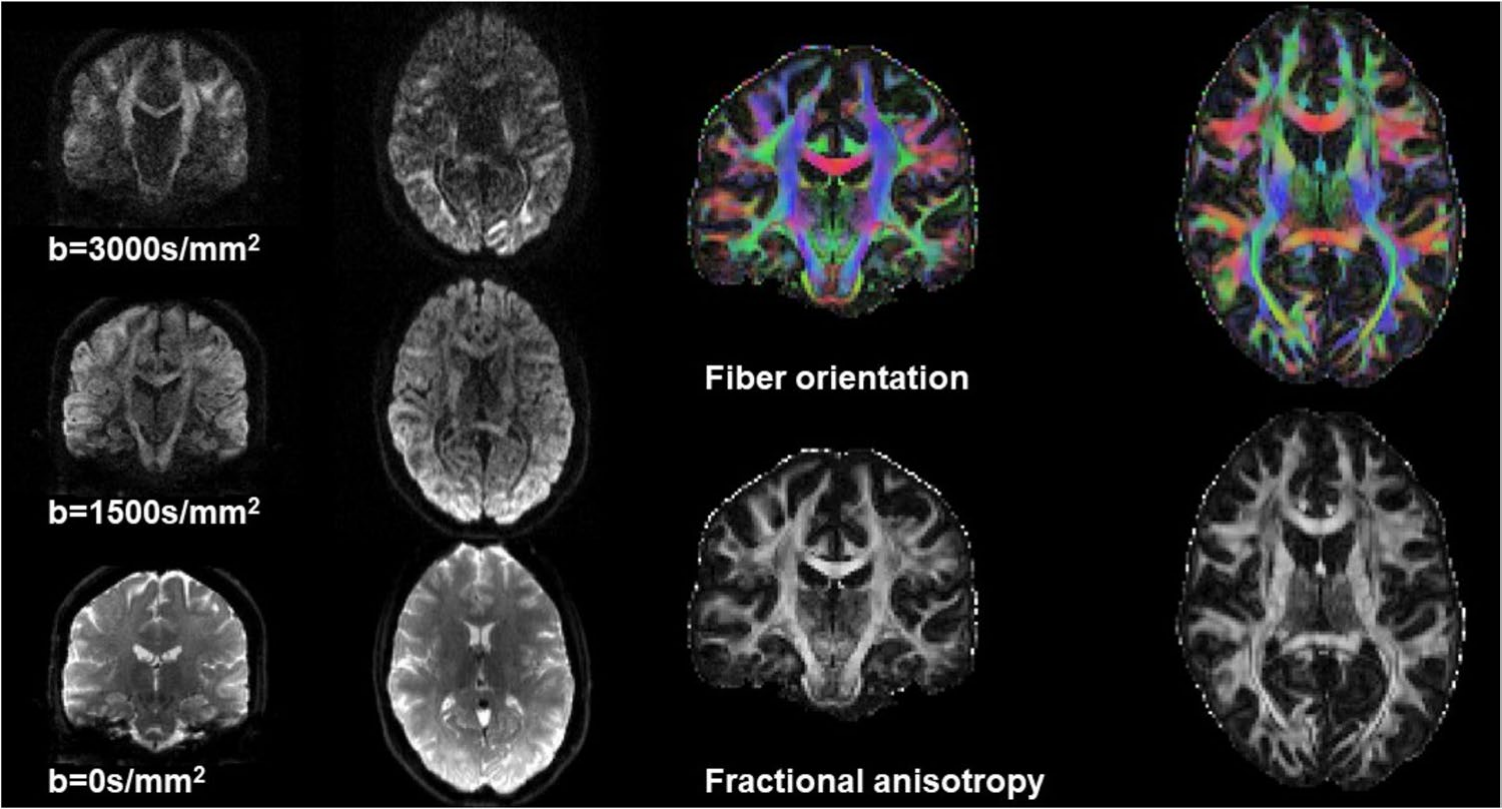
Example diffusion MRI data obtained with the recommended MRI protocol. The left panel shows examples of *b* = 0 s/mm^2^, *b* = 1500 s/mm^2^, and *b* = 3000 s/mm^2^ images after preprocessing. The right panel shows the corresponding fractional anisotropy map and primary fiber orientation from the diffusion tensor
